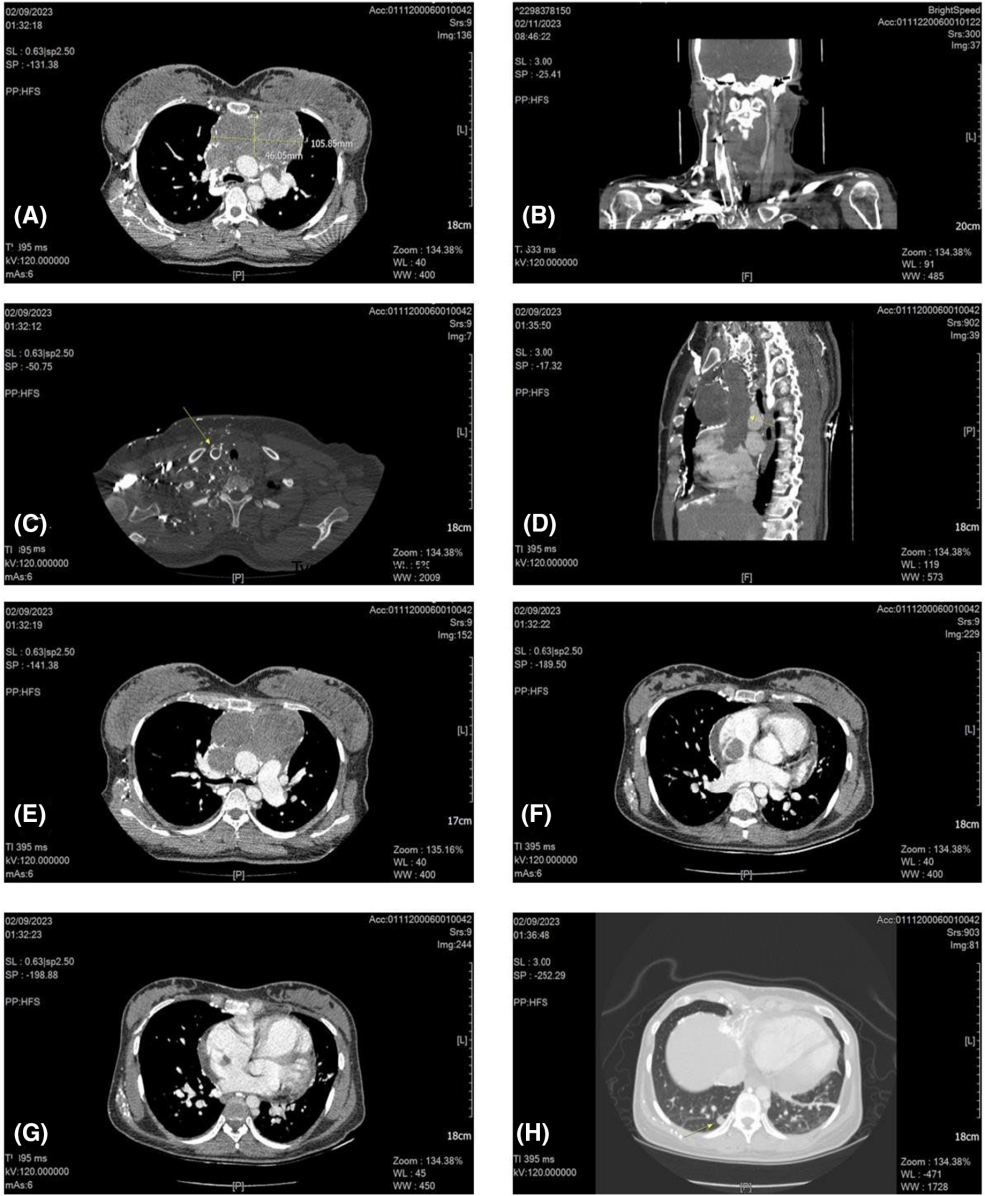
Pulmonary CT angiography. Evidence of a heterogeneous lobulated hypo‐dense mass measuring 105 × 6 mm is seen at the anterior mediastinum (A). Evidence of extension of tumor thrombosis into the right jugular vein is seen (B, C). Evidence of extension of tumor thrombosis into the superior vena cava is seen (D). Evidence of a large heterogeneous hypo‐dense lobulated mass extending to the superior vena cava is seen (E). Extension of tumor thrombosis from the superior vena cava into the right atrium is seen (F). Evidence of ASD is present (G). Evidence of multiple lung nodules up to 10 ×9 mm in the basal segment of the right lower lobe due to lung metastasis is seen in both lung fields (H).
